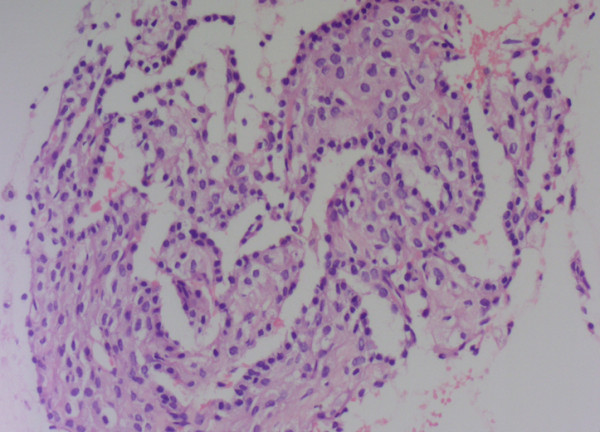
Pulmonary sclerosing hemangioma was confirmed by CT-guided percutaneous lung biopsy postoperative pathological diagnosis. Papillary areas: cuboidal cells were on the papillary surface and polygonal cells were in the stroma (×200).
